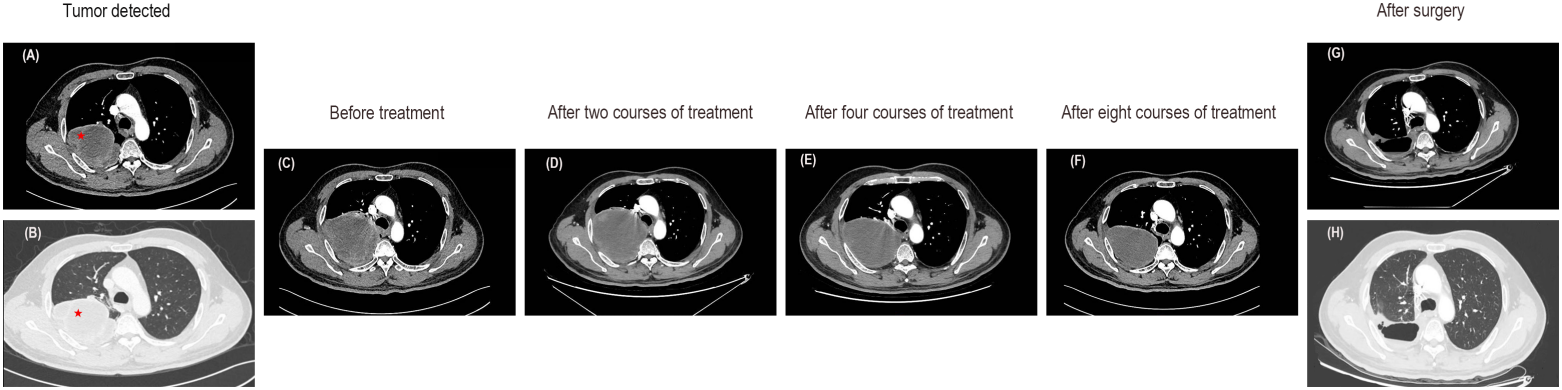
Enhanced CT images of the lung mass. (A, B) CT images at the time of tumor detection. (C) The CT images before any treatment. (D) Enhanced CT scans after two courses of treatment indicated a slightly enlarged tumor with an overall stable efficacy. (E) A subsequent CT scan after the fourth course of treatment showed stable disease. (F) The CT images after eight courses of treatment suggested a decrease in tumor size. (G, H) Enhanced CT revealed no tumor recurrence after surgery.
